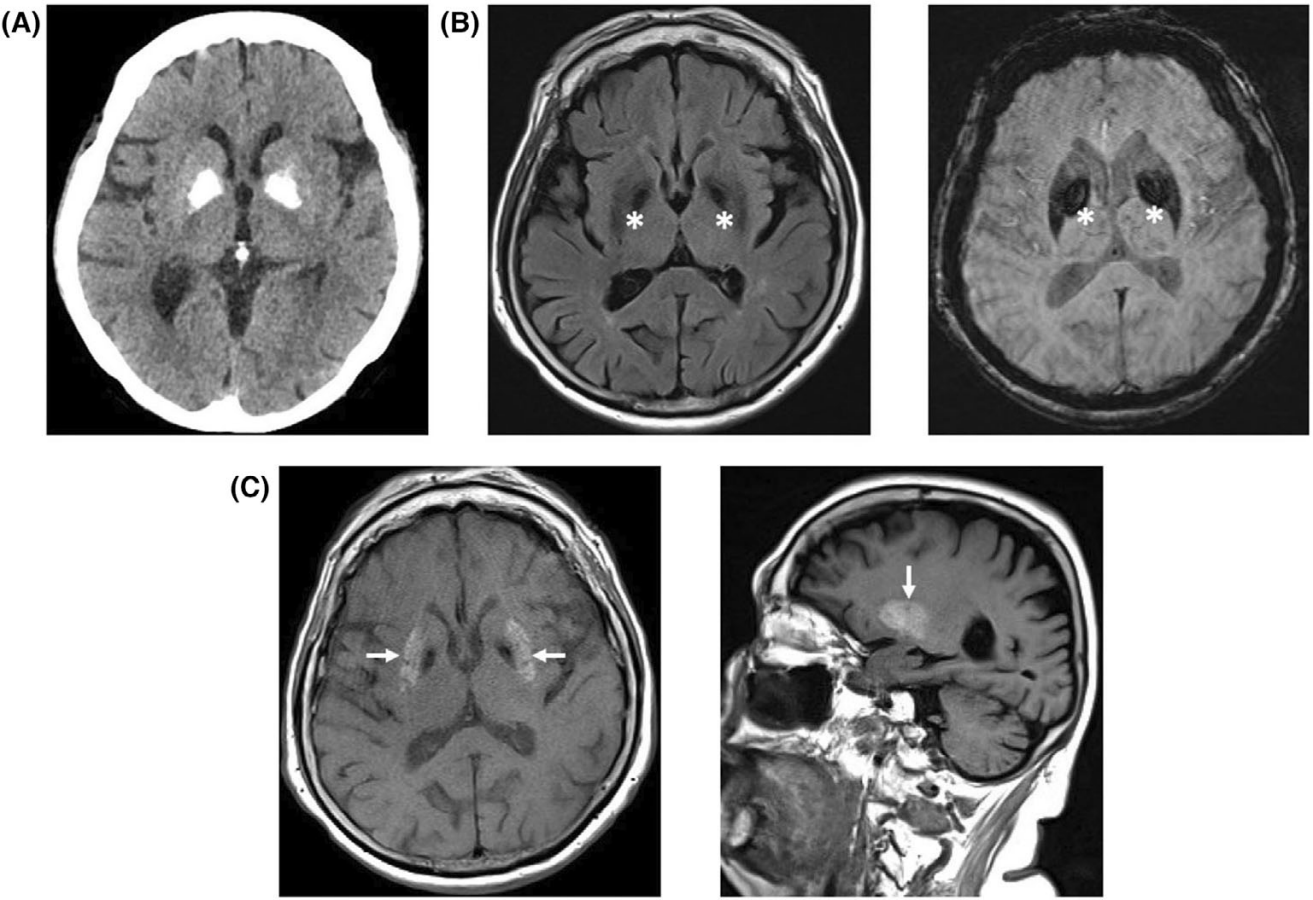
CT head and MRI brain showing globus pallidus calcifications and putaminal T1 hyperintensity. (A) Non‐contrast head CT axial image showing bilateral hyperdensities in the globus pallidi consistent with age‐related calcification, unchanged from CT head 1 year prior (not shown). (B) MRI brain axial T2 FLAIR sequence (left) showing T2 hypointensities in bilateral globus pallidi (indicated by white asterisk) corresponding to hyperdensities on CT; SWI sequence (right) showing susceptibility artifact in bilateral globus pallidi (indicated by white asterisk) corresponding to CT hyperdensities, consistent with age‐related calcification. (C) MRI brain axial (left) and sagittal (right) T1 pre‐contrast sequences showing hyperintensity of bilateral putamen (indicated by white arrows).
